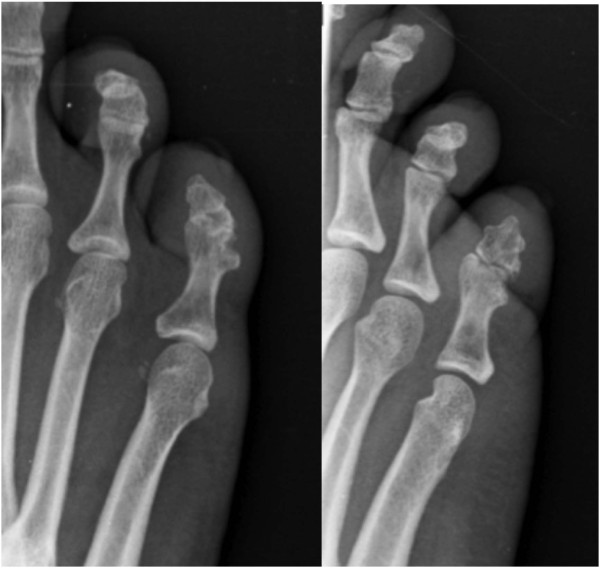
Right fifth toe: Radiograph 12-month post-surgery, showing the joint congruity and no evidence of arthritic changes.
